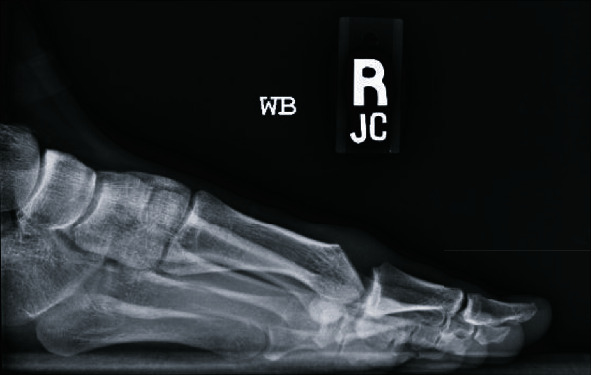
Preoperative right foot lateral radiograph taken at the time of presentation showing prior dorsal cheilectomy.
